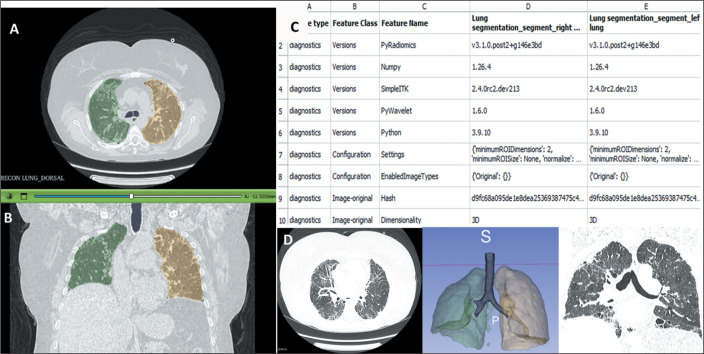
Radiomic analysis of HRCT using the software 3D Slicer, version 5.8.1
